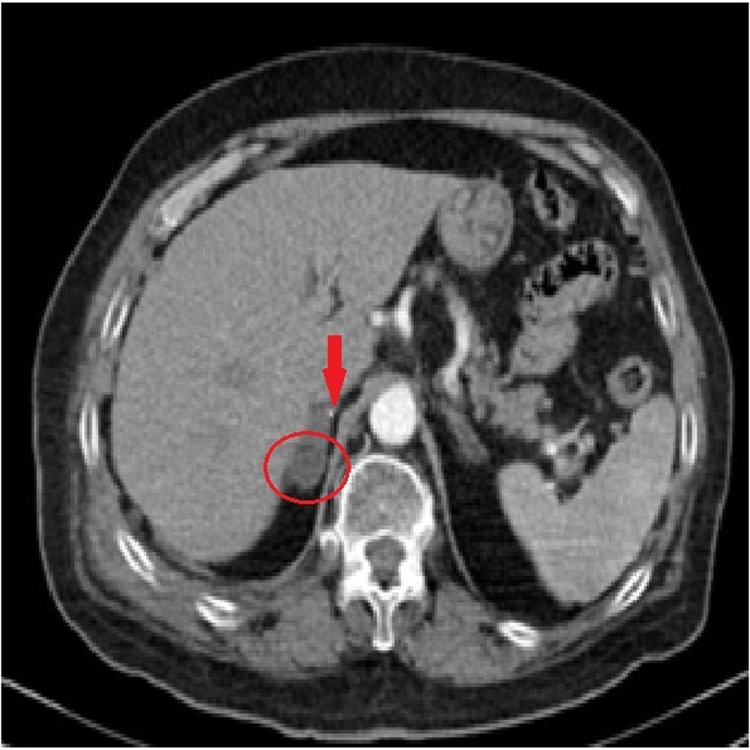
CE-CT reveals a contrast-enhanced dot-shaped focal area anterior to the gland (arrow).
